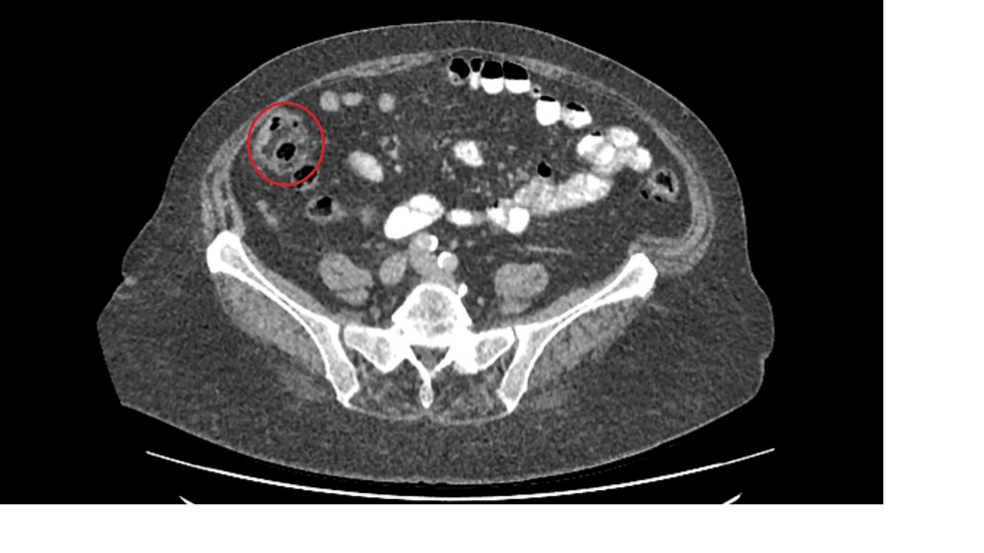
Portal venous phase CT abdomen - axial view showing circumferential caecal wall thickening
